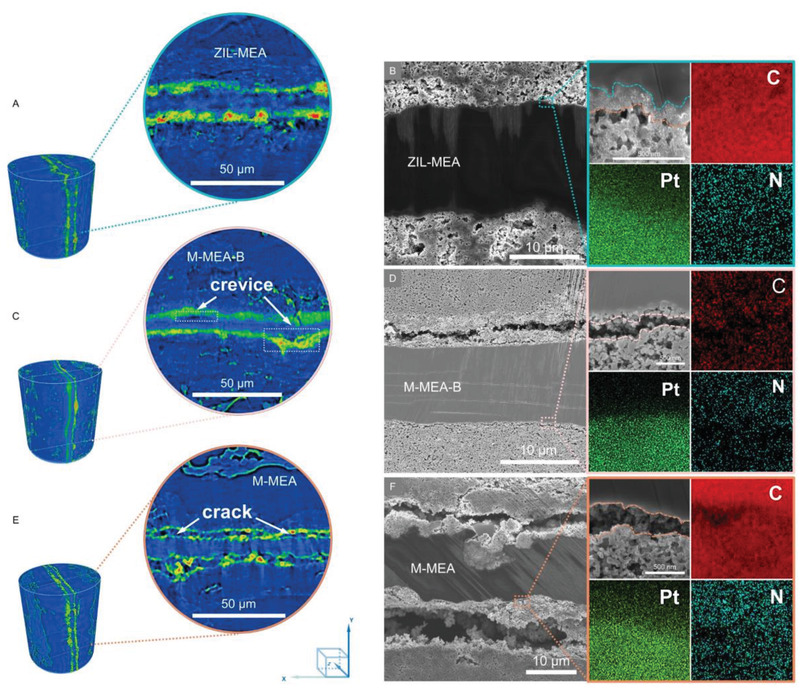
The resulting changes in the MEAs after initial constant RH durability testing. A,C,E) Micro‐CT images of ZIL‐MEA (A), M‐MEA‐B (C), and M‐MEA (E) and B,D,F) cross‐section SEM images coupled with EDX for ZIL‐MEA (B), M‐MEA‐B (D), and M‐MEA (F), all recorded after the constant current discharge durability test.
